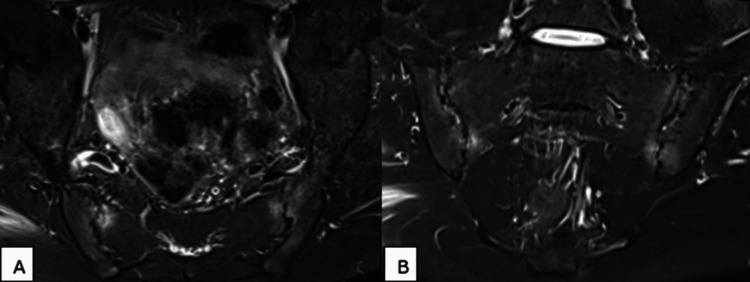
(A) Axial and (B) coronal MRI in STIR sequence of the sacroiliac joints revealing subchondral bone marrow edema with associated erosions
